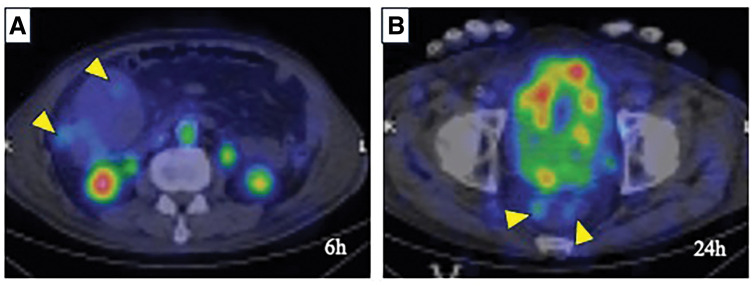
^99m^Tc-labeled HSA scintigraphy. (A) At 6 h, protein leakage is observed within the tumor (arrowhead). (B) At 24 h, the leaked protein is seen flowing into the rectum (arrowhead). HSA, human serum albumin
